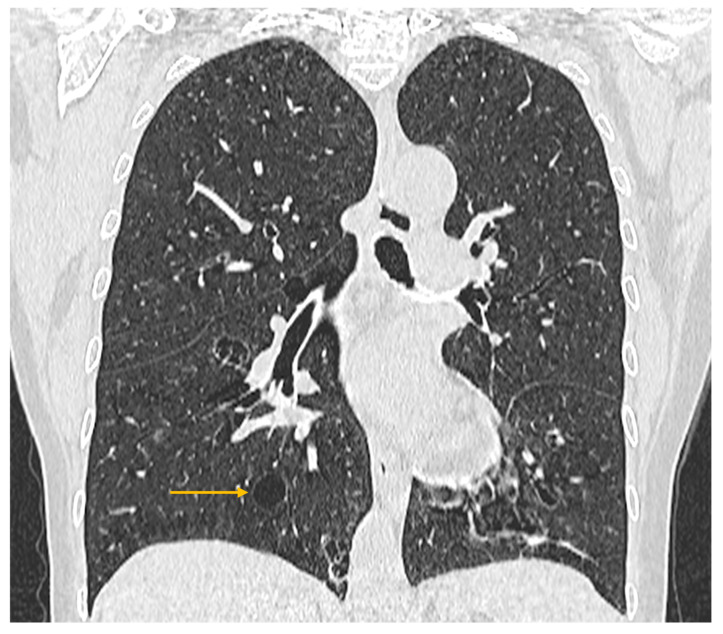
High-resolution computed tomography (HRCT) of the chest in lymphocytic interstitial pneumonia (LIP). Coronal HRCT image demonstrates thin-walled pulmonary cysts (arrow), a characteristic imaging feature of LIP.
